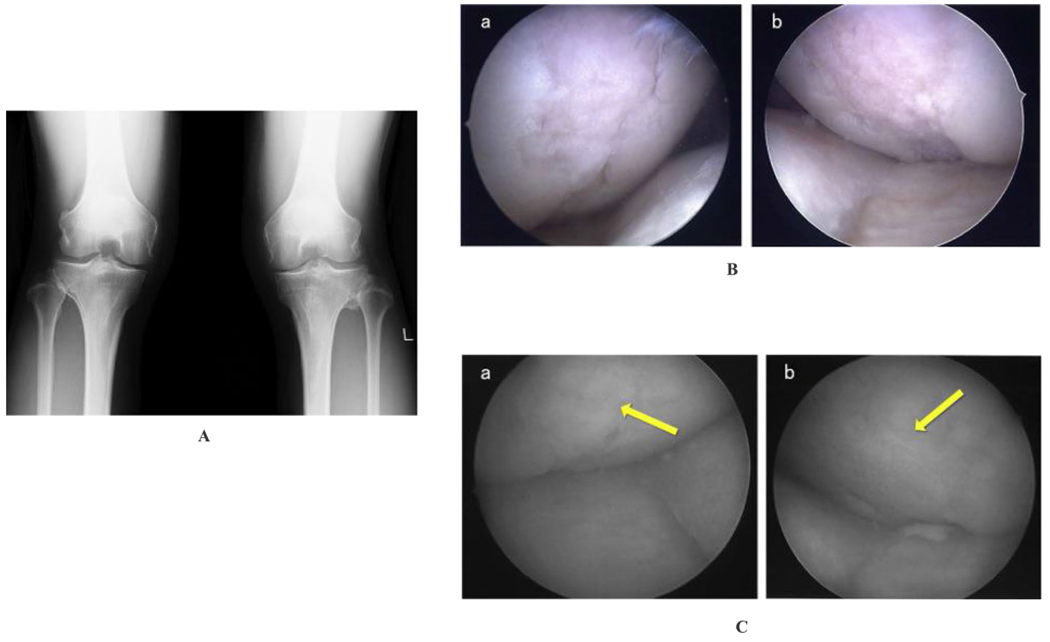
A X-ray was taken in a weighted postero-anterior view of the knee at 45° flexion (Rosenberg’s view), showing a slight and moderate medial joint space narrowing in the right and left knee, respectively (KL grades II, III). B Preoperative arthroscopic findings in the right and left medial femoral condyles show 2.5 × 1.5 cm cartilage defect in the right knee (a), and 3 × 1.5 cm cartilage defect in the left knee (b). C Second-look arthroscopic findings in the right and left medial femoral condyles 6 months post-operation revealed that the cartilage defect area was covered by regenerated cartilage. ((a) right), ((b) left). Adapted from Yuma Onoi et al., 2019 [8]
